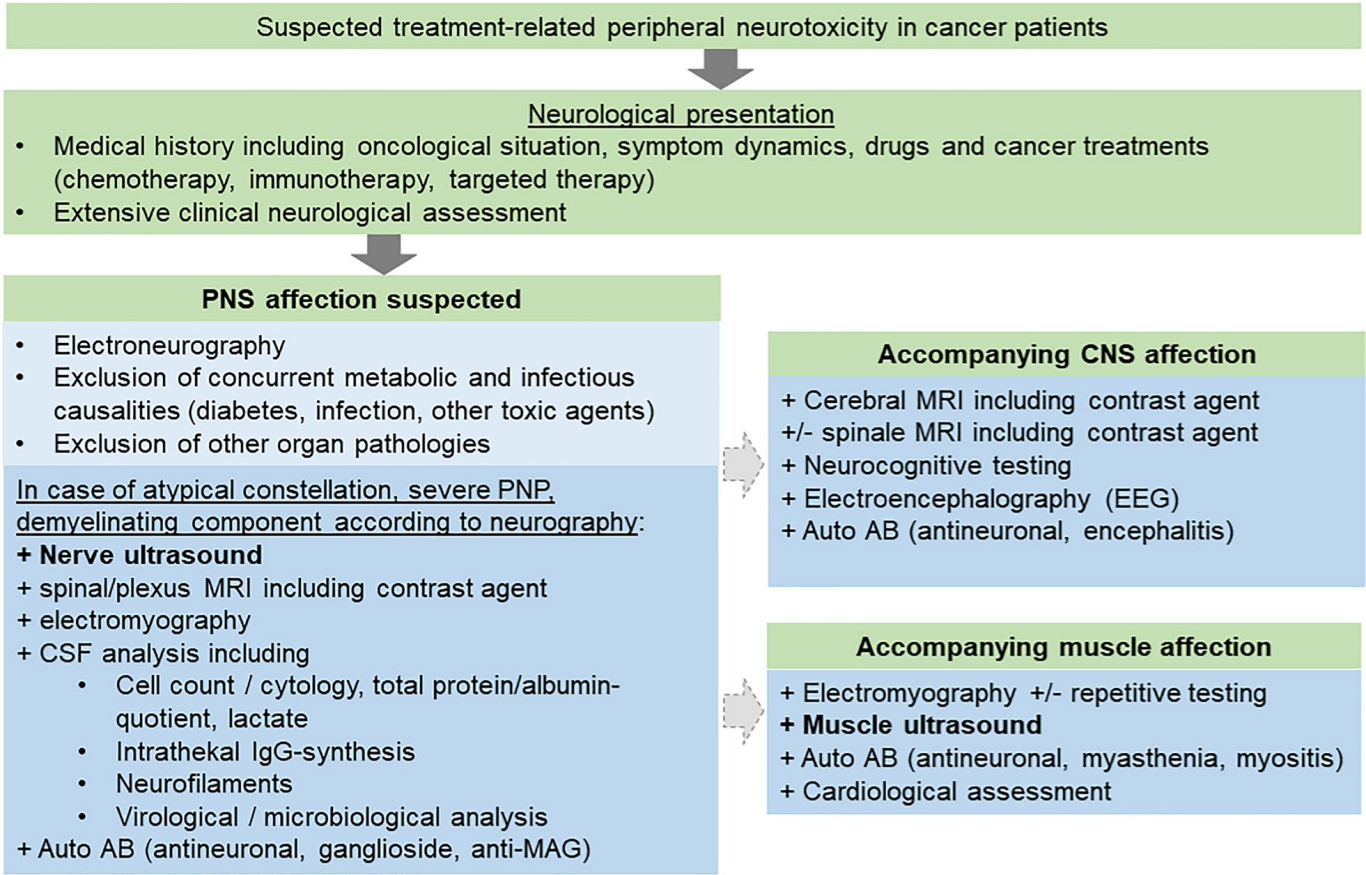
Diagnostic algorithm for cancer patients presenting with suspected treatment-related peripheral neurotoxicity. The figure shows our propose for a standardized diagnostic algorithm including NMUS as a part of routine neurological assessment for cancer patients with suspected peripheral neurotoxicity. Abbreviations: *CNS* central nervous system, *CSF* cerebrospinal fluid, *MRI* magnet resonance imaging, *IgG* immune globulin G, *Auto AB* auto antibodies, *anti MAG* antibodies against myelin-associated glycoprotein, *PNP* polyneuropathy, *PNS* peripheral nervous system
